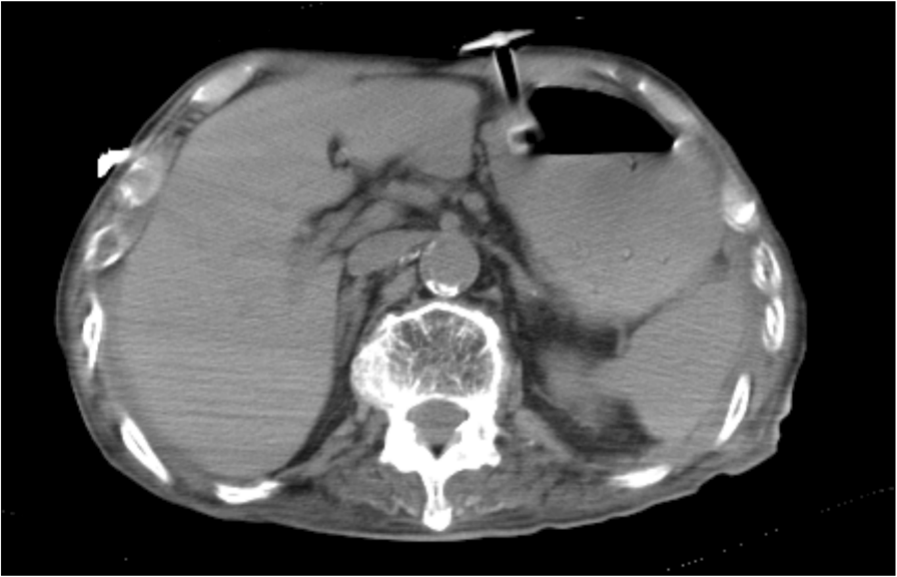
Computed tomography obtained 3 weeks before admission with diagnosis of aspiration pneumonia showed no hepatic portal venous gas and no gas within the wall of stomach in the visualized portions of the upper abdomen
